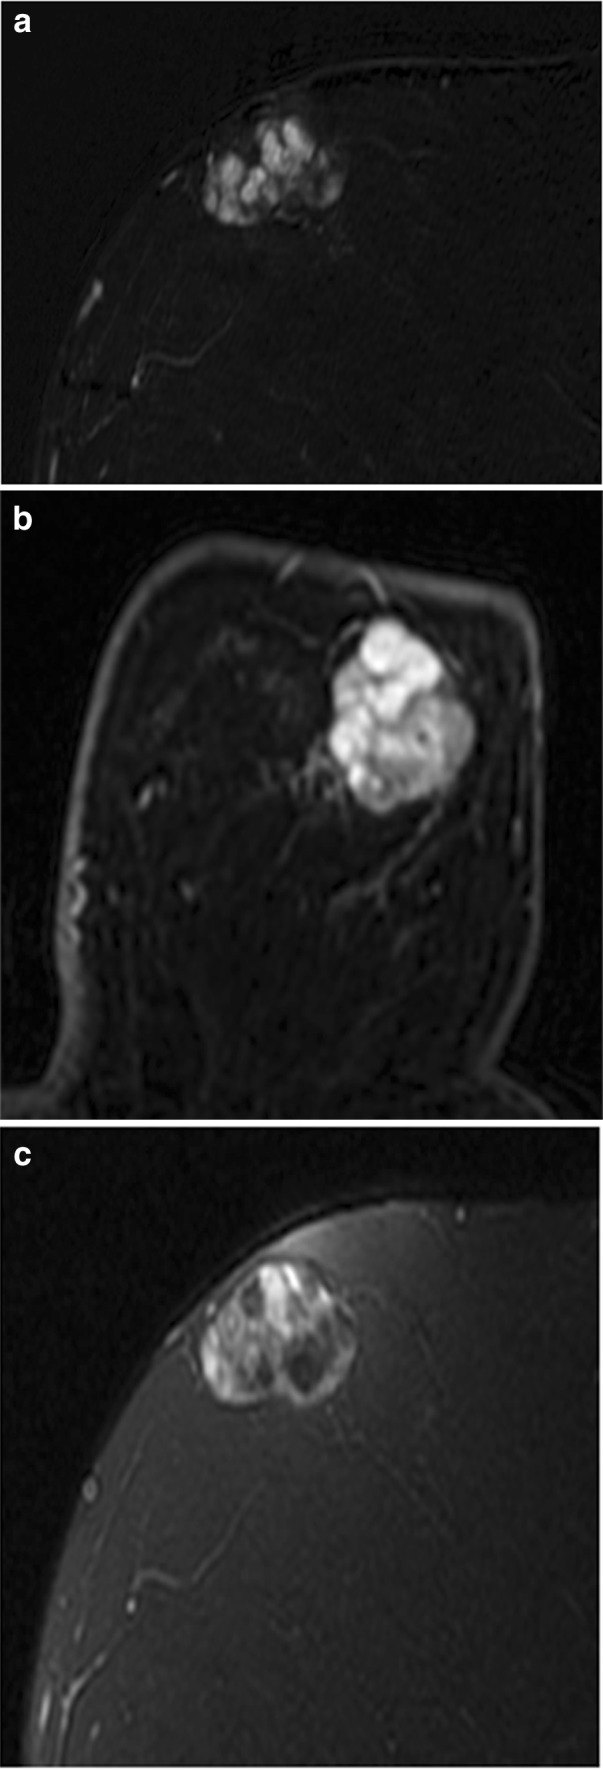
Fibroadenoma. Postcontrast subtraction T1-weighted sagittal (a) and axial (b) images show a 3.6-cm oval mass with circumscribed margins and dark internal septations. On c fat-saturated T2-weighted image, it demonstrated high signal intensity and is most consistent with a fibroadenoma. If this mass was an incidental finding on baseline MRI, a BI-RADS 3 assessment would be appropriate
